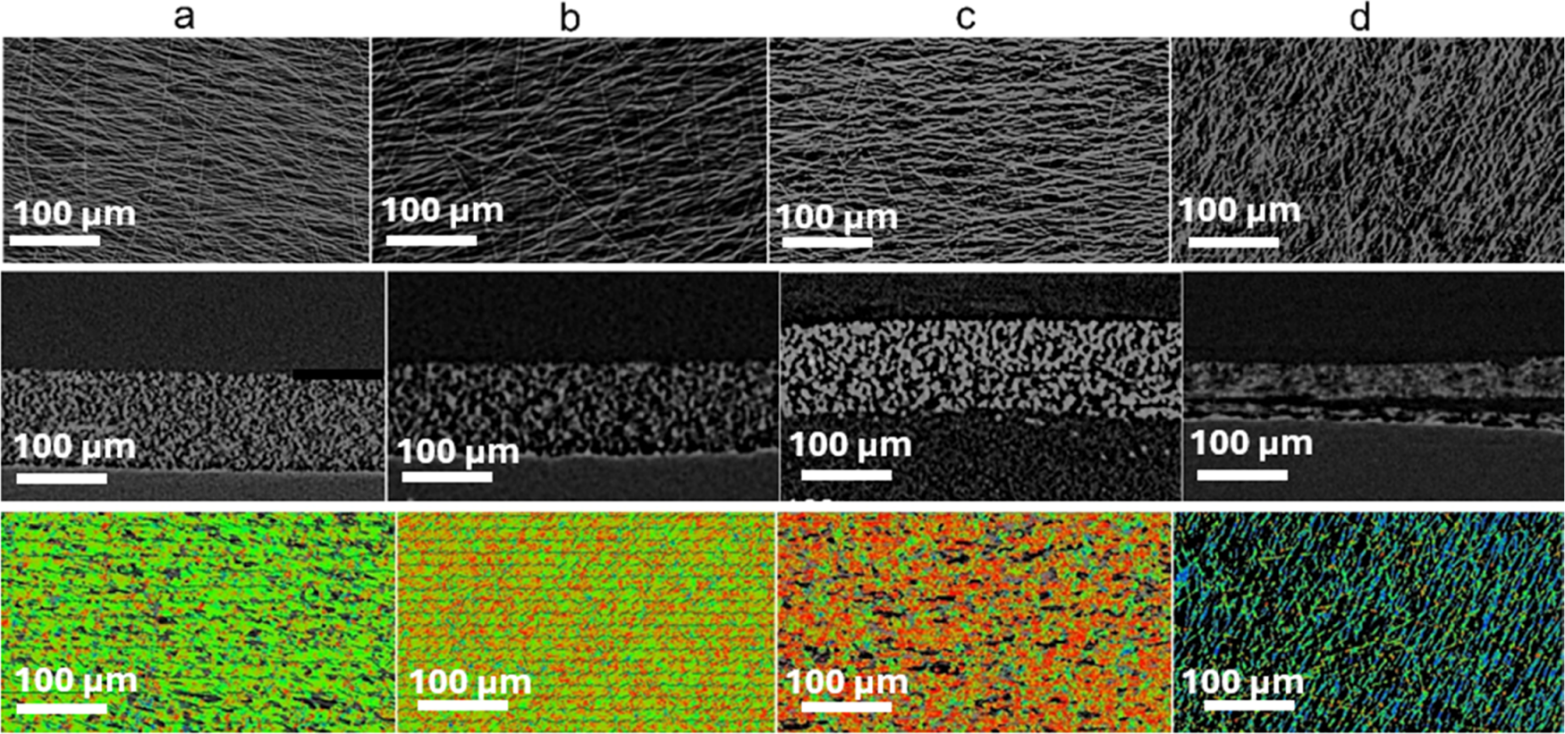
X-ray computed tomography
